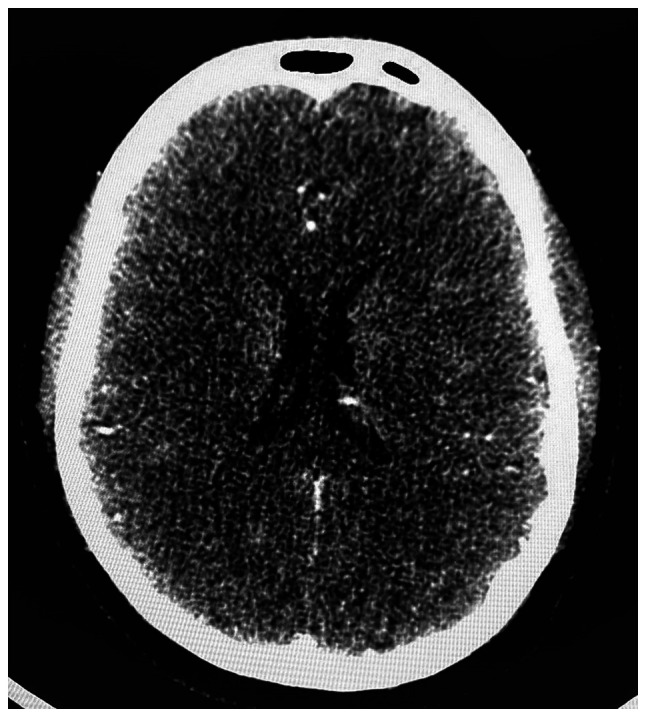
Native head computed tomography scan.
